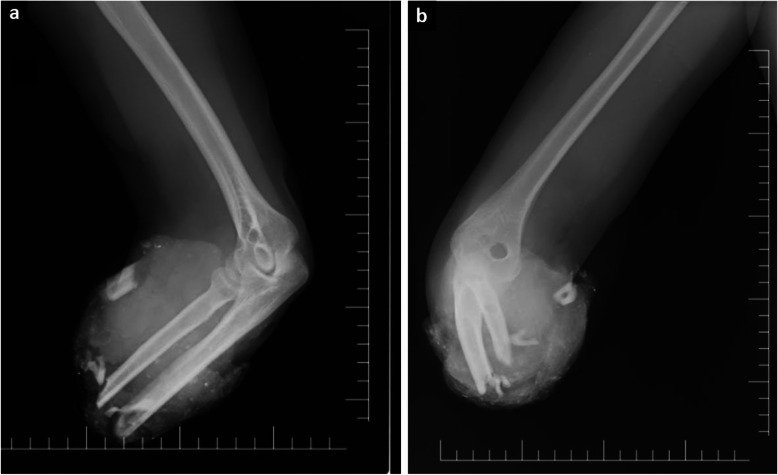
Radiography images of an 18-year-old woman with traumatic amputation. a-b Anteroposterior and lateral radiographs showing transradial traumatic amputation, type 3C open fractures in the radius and ulna, extensive soft tissue loss, and fragmented bones
